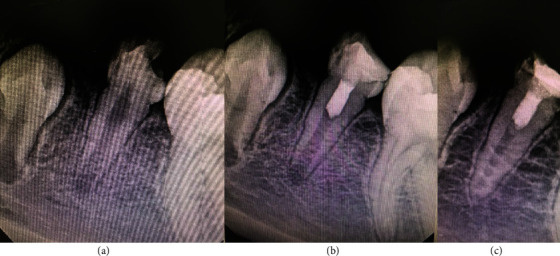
Permanent tooth 35. The image (a) shows diagnostic X-ray, image (b) immediate postoperative, and image (c) control at 24 months.
